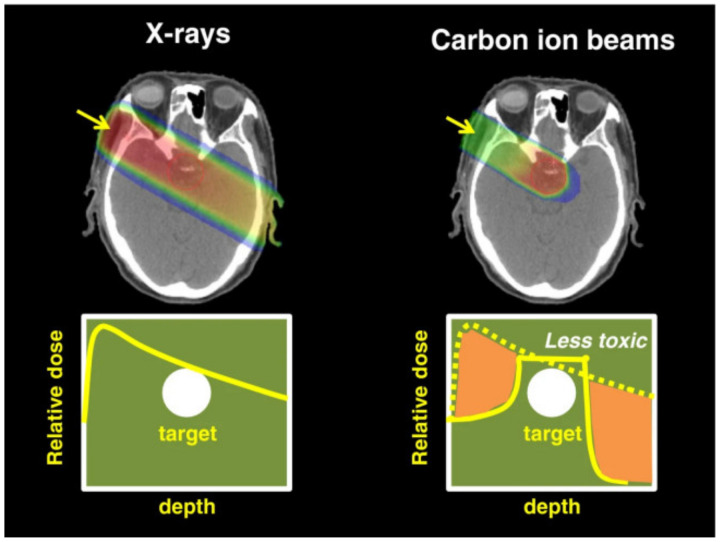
The advantage of carbon ion beams compared with X-rays through a better dose distribution. As a result of the “Bragg Peak” effect mentioned above, carbon ion beams allow a highly localized deposition of energy, which can be utilized for increasing radiation doses to tumors while minimizing irradiation to adjacent normal tissues (personalized cancer treatment). Reproduced with the permission from Ohno, EPMA J.; published by Spring, 2013.
